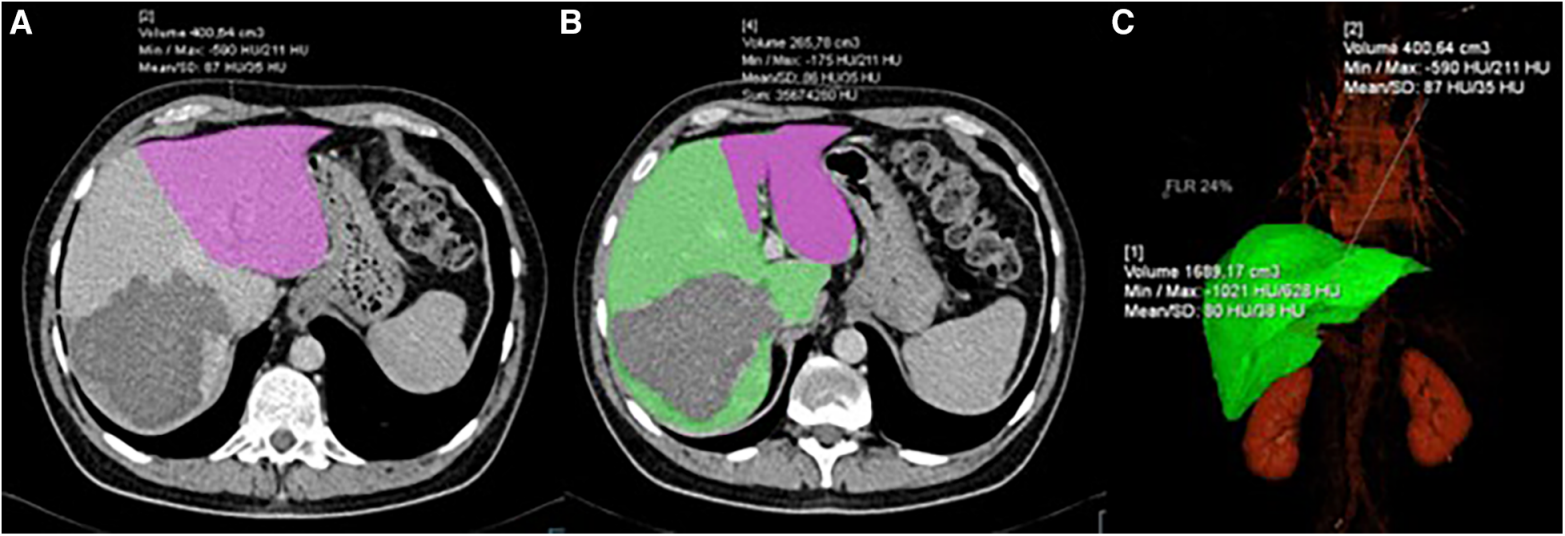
CT volumetry process showing: (A) delineation and volume calculation of FLR (pink); (B) delineation of FLR (pink) and DPL (green); (C) 3D reconstruction of the liver with volume calculation.
